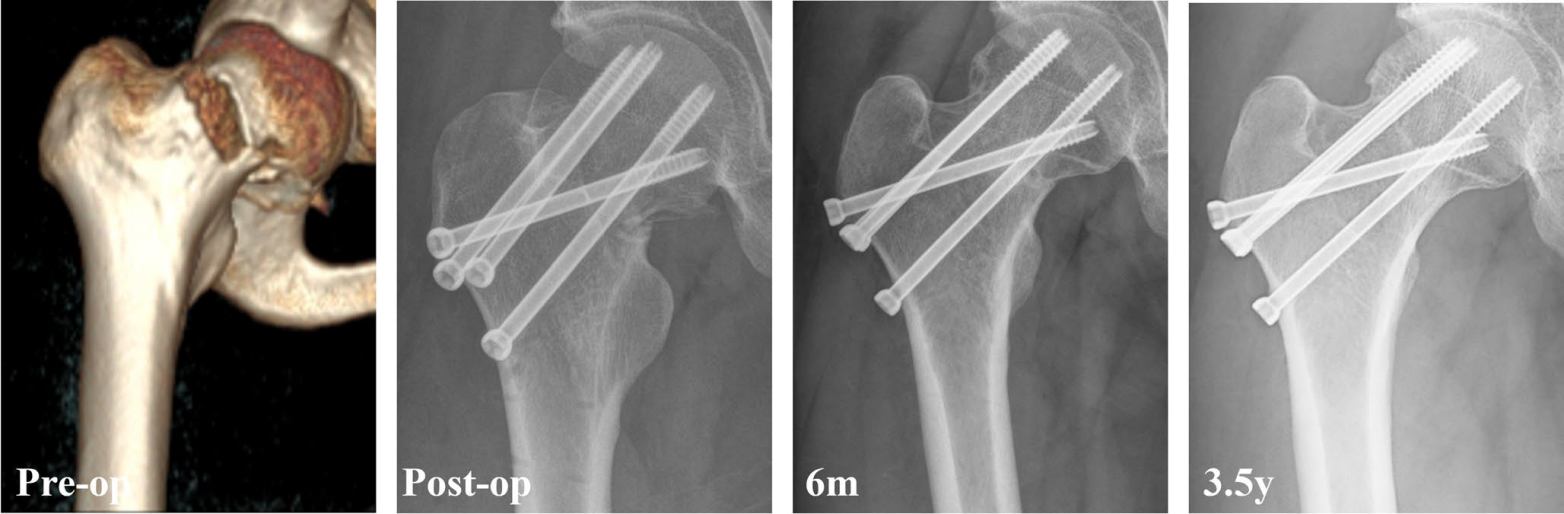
A 44-year-old male who sustained a Pauwels III femoral neck fracture was treated with alpha fixation. The radiograph taken 6 months and 2 years postoperatively revealed fracture union without complications
